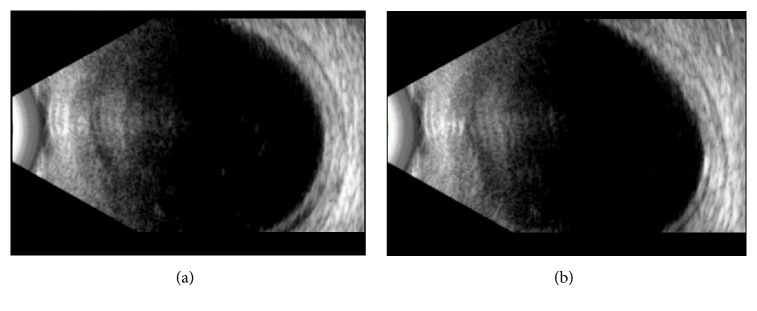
Mode B ultrasonography showing choroidal effusion at diagnosis ((a) right eye; (b) left eye).
